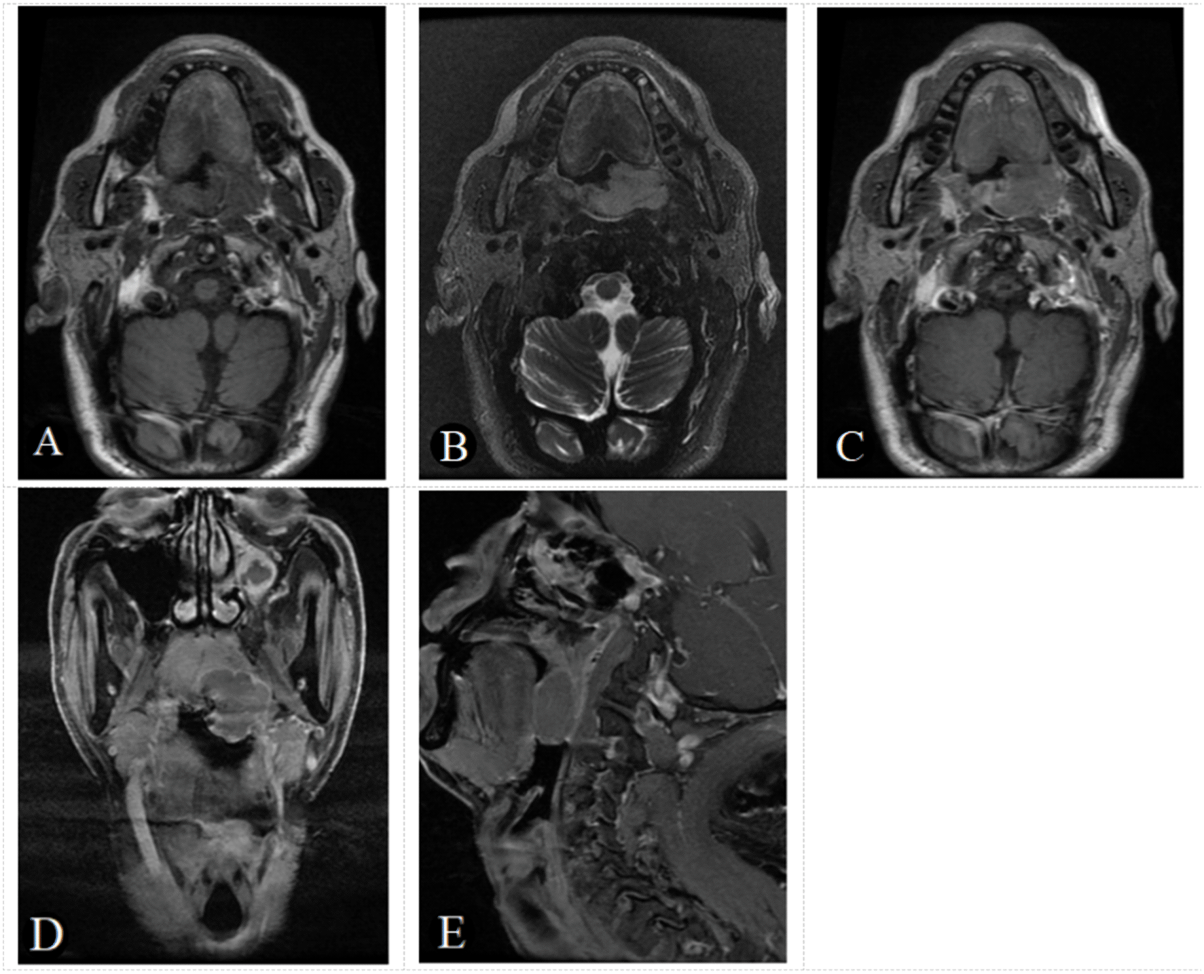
A 61-year-old male patient with negative EB virus nucleic acid and DLBCL in the left oropharynx. The tumor appears as a homogeneous focal nodular with an isointense signal on axial T1-weighted image (A), a slightly hyperintense signal on T2-weighted image (B) and a slightly homogeneous enhancement, on axial, coronal, sagittal enhancement T1-weighted image (C-E). The tumor boundaries are clear and there is no bone destruction. Based on the score model, this patients scored 0 points, the minimum in the points-scoring system.
